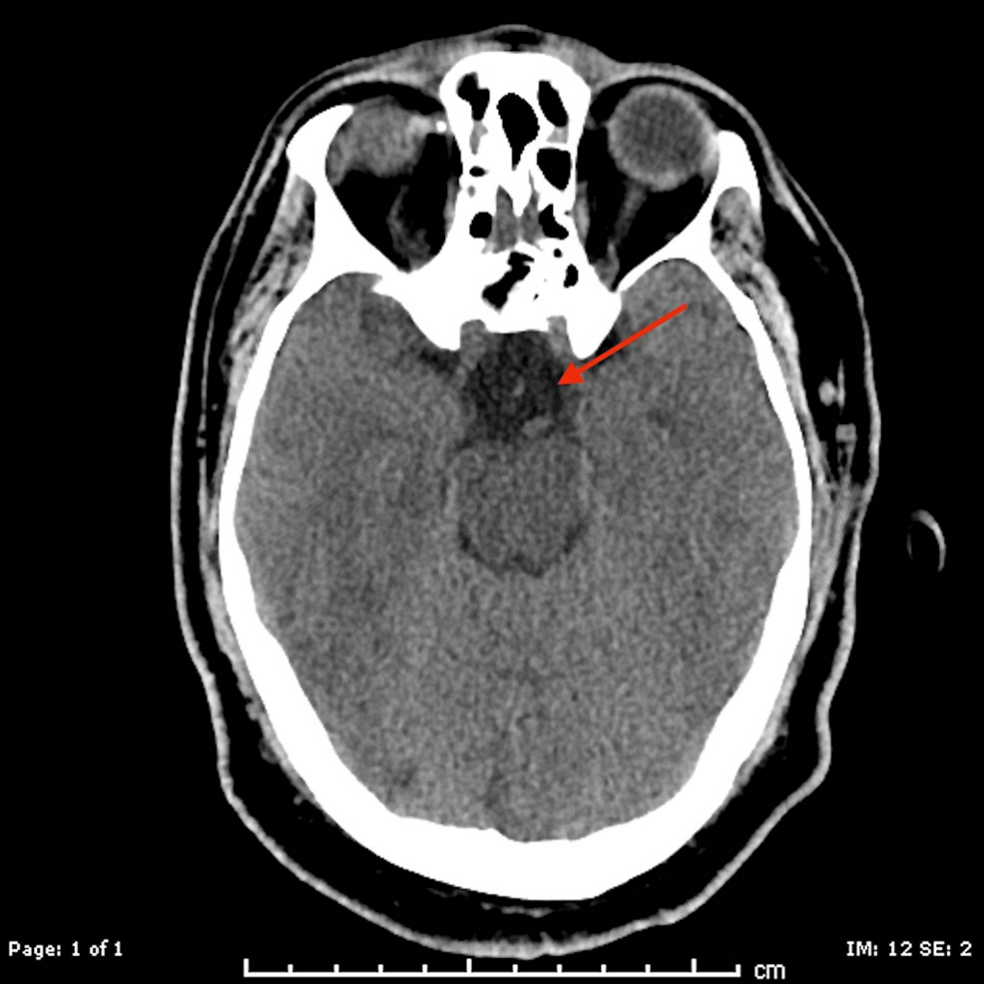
CT head was taken after transsphenoidal resection of the pituitary mass. The arrow shows the interval removal of pituitary mass with no unexpected post-operative changes. CT: computed tomography
